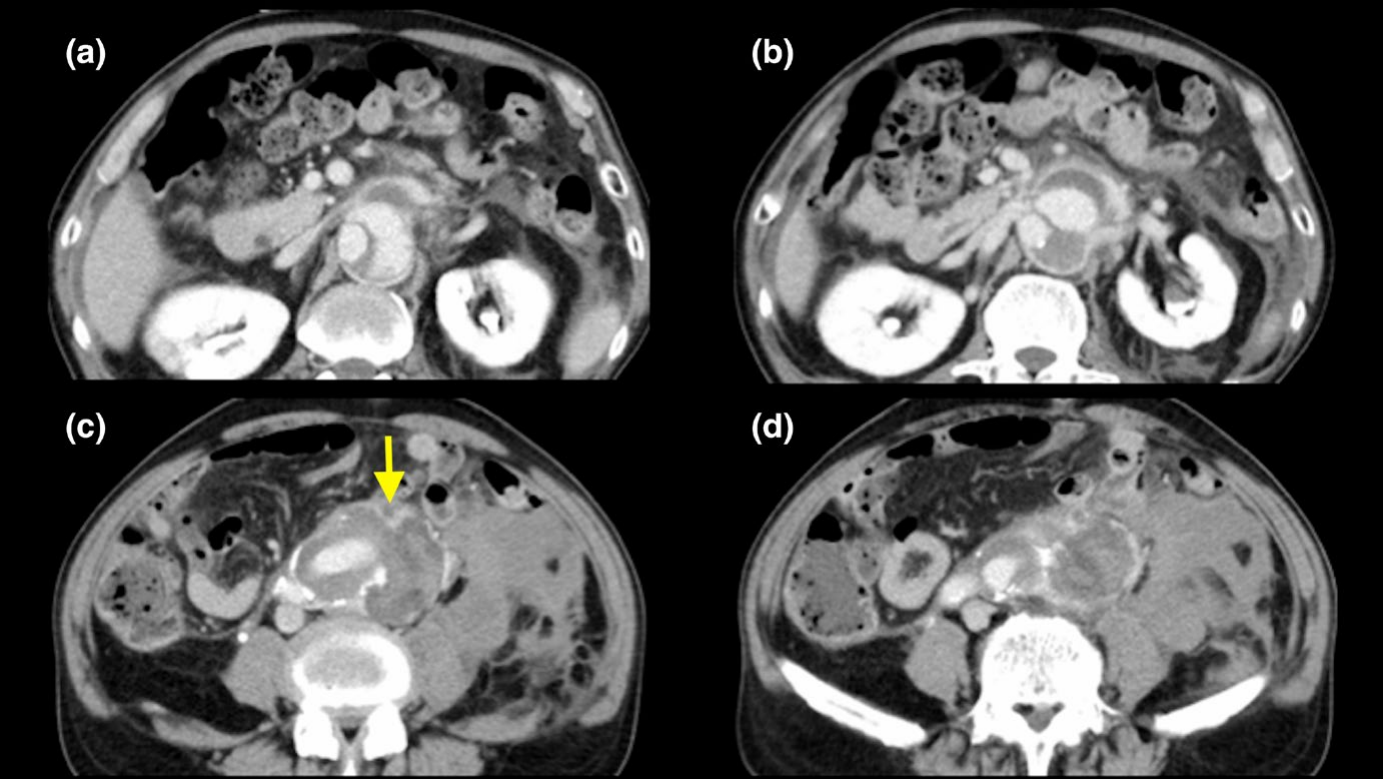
Computed tomography angiogram reveals a rupture of the adventitia (yellow arrow) and a hematoma in the retroperitoneal cavity. (A) At the level of the superior mesenteric artery, (B) at the level of the renal arteries, (C) infrarenal level, and (D) at the level of the terminal aorta. This computed tomography angiogram was obtained 1 week after the computed tomography angiogram (Figure 1) was taken
